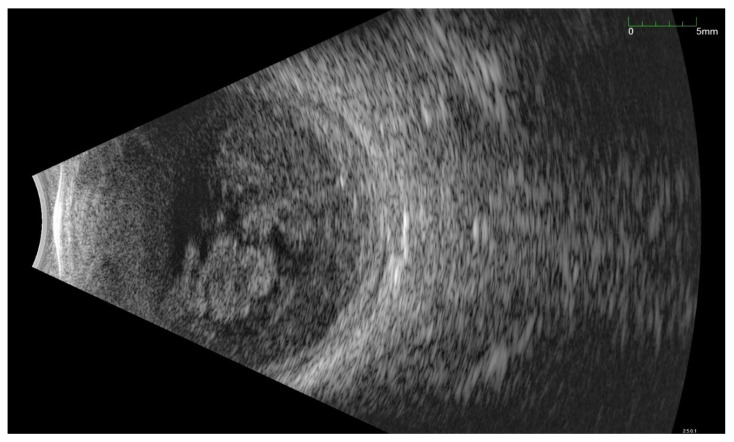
Ultrasound B scan with a hyperechoic exudate filling almost the entire vitreous chamber. Advanced inflammation in a patient with bacterial EE.
